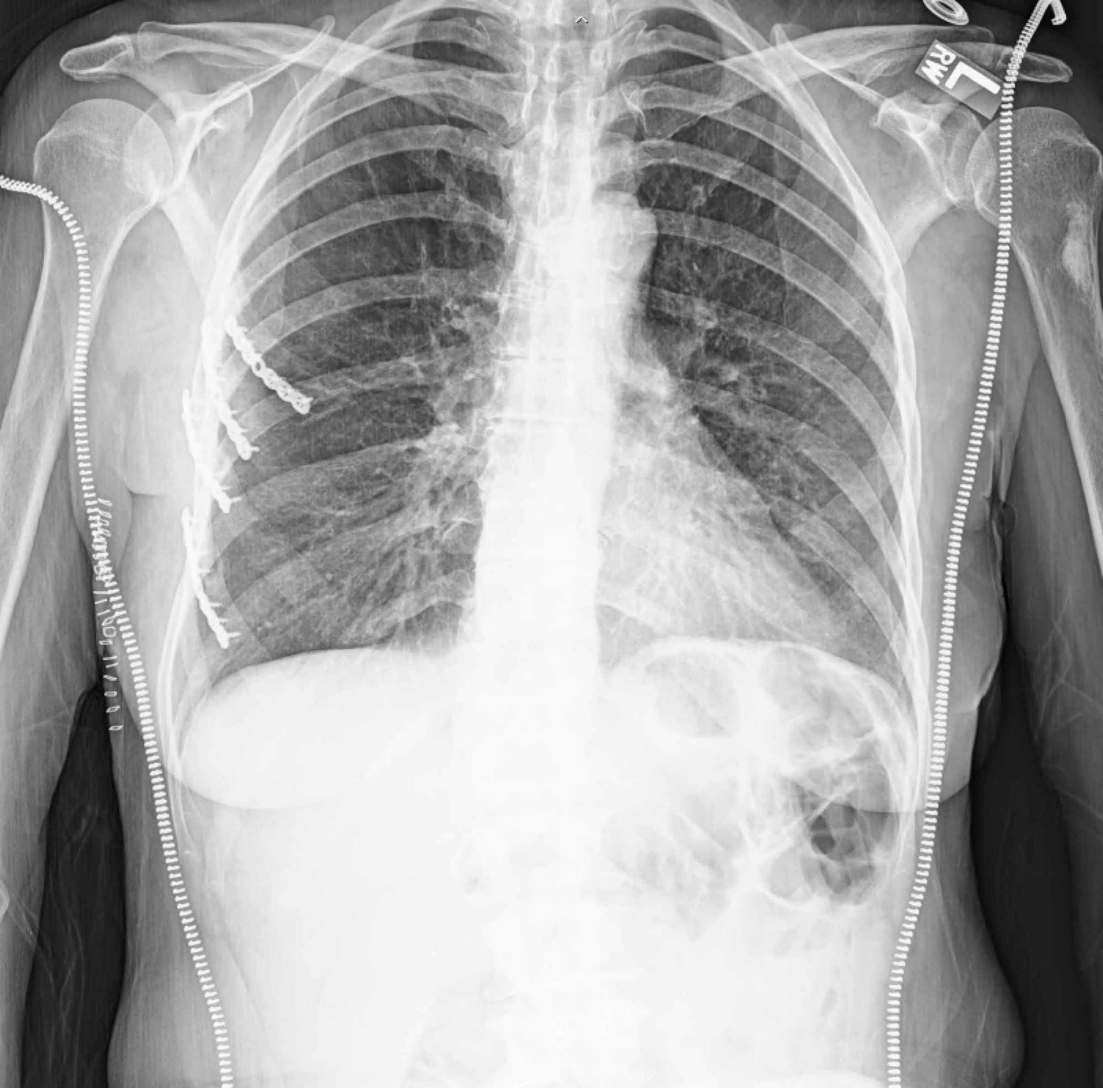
Final Radiograph Fully resolved pneumothorax and subcutaneous emphysema; rib fractures have also gone onto successful union.
